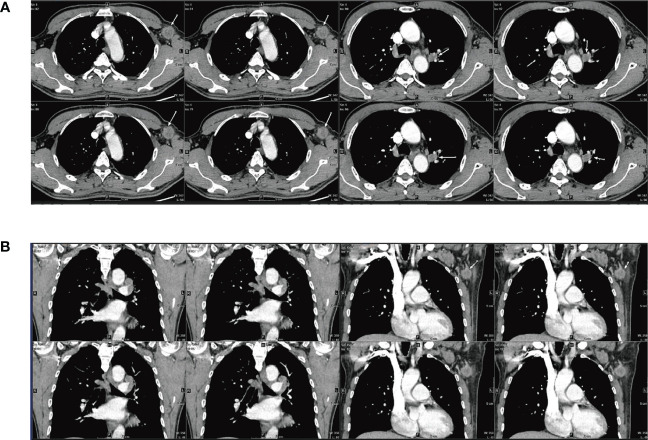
CT and MRI scan results for the patient (A) CT: Soft tissue nodular mass in the left upper lung adjacent to the mediastinum, measuring approximately 1.31×1.79 cm; multiple masses and enlarged lymph nodes in the aortopulmonary window, above the eminence, and both lung hilums; larger mass at the left lung hilum measuring 1.29×2.06 cm; (3) Mass with slight density visible in the left axilla, unevenly enhanced nodule is visible, measuring approximately 2.32×2.5 cm. (B) MRI: Dynamic contrast-enhanced MRI: multiple masses and enlarged lymph nodes in the aortopulmonary window, above the eminence, and both lung hilums; mass in the left lung hilum measuring approximately 1.6×1.6 cm; Enlarged lymph nodes visible in the left axilla, measuring approximately 2.4×1.9 cm.
